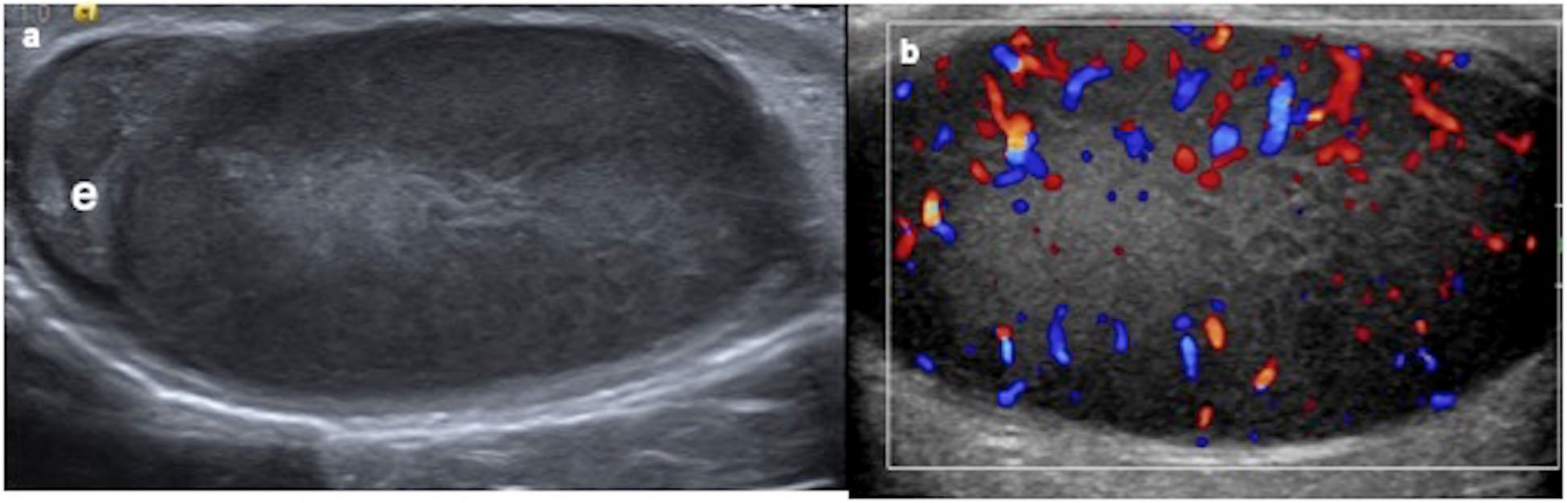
Testicular leukemic infiltration. A 8-year-old boy diagnosed of leukaemia with a right swollen testis. a Longitudinal ultrasound view shows an enlarged and diffuse hypoechoic right testis. e (epididymis). b Colour Doppler demonstrates increased vascularization
